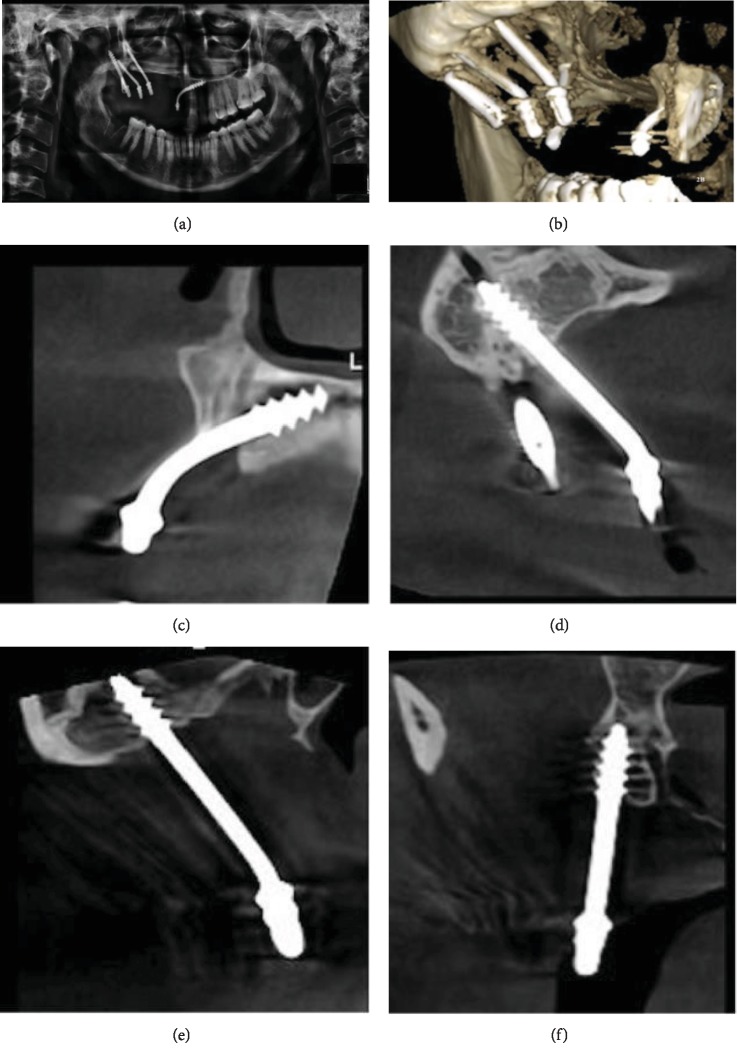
(a) Postoperative panoramic view showing that the overerupted 47 had been extracted to avoid unwanted and noncontrollable further eruption, which could damage the implant's integration through mechanical overload. In addition to the lake of the opposing artificial teeth occlusion (the prosthesis has only an upper first molar) to eliminate the cantilever force and to reduce and ensure better force distribution. (b–f) A cone beam computer tomography showing a 3-dimension view for the implants' positions; one in the contralateral nasal floor, two implants at the pterygoid plates, and one implant at the zygomatic bone.
